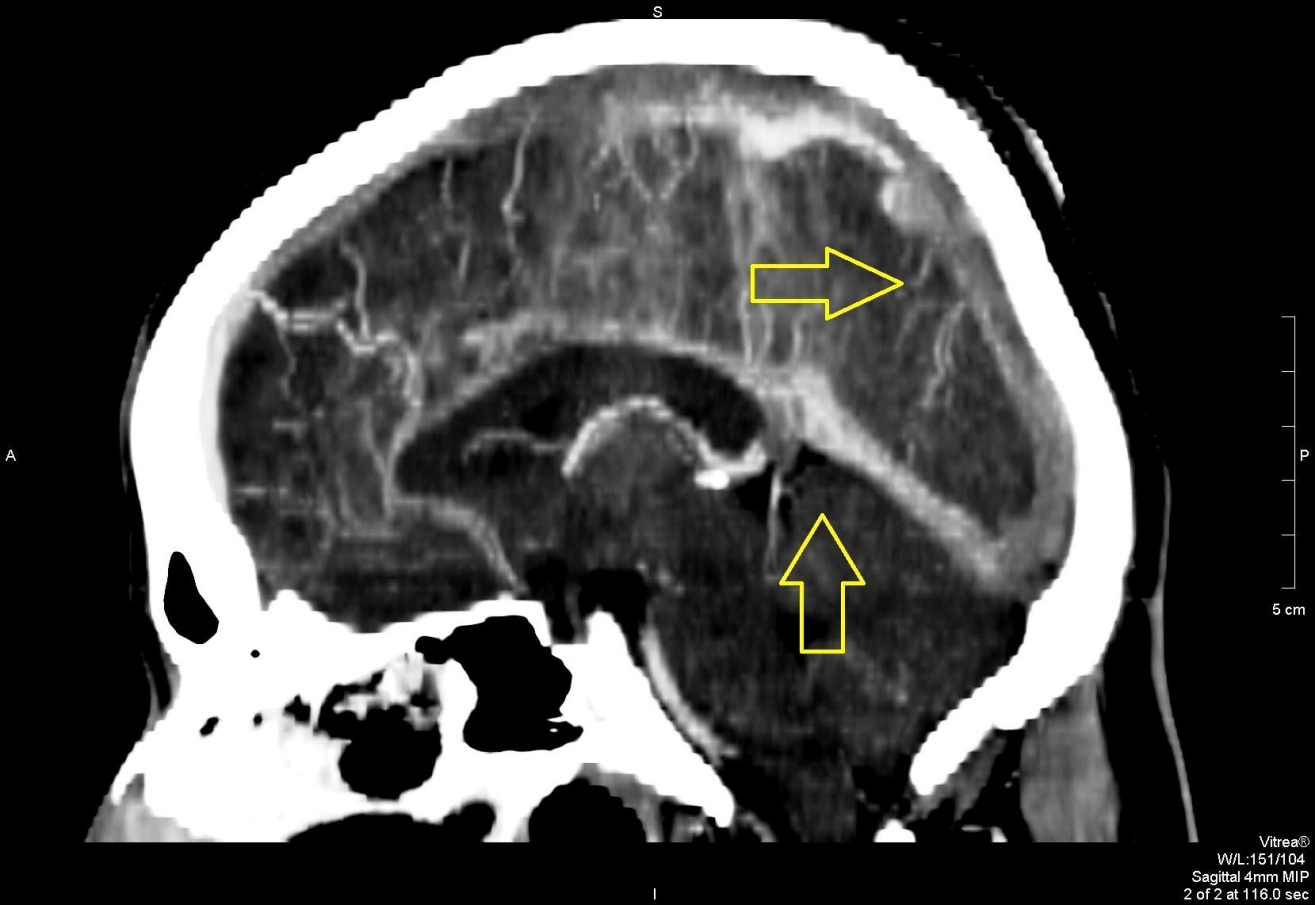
Sagittal view on contrast CT scan showing extensive sinus blockage CT: computed tomography
